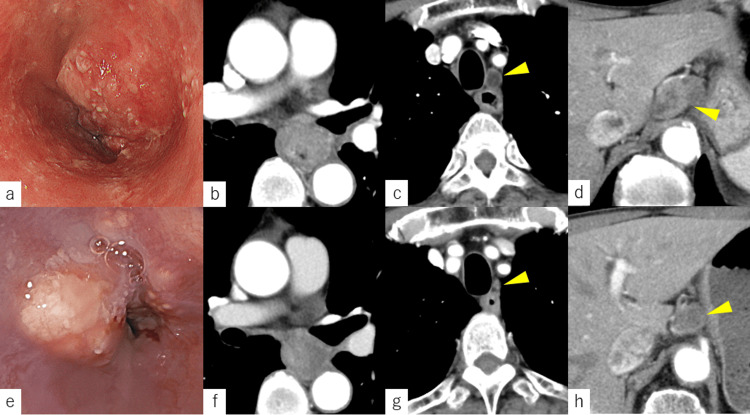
Images from upper gastrointestinal endoscopy and computed tomography (CT) Before NAC: (a) Upper gastrointestinal endoscopy shows an advanced tumor with stenosis; (b) CT shows the advanced cancer in the middle thoracic esophagus; (c) CT shows the lymph node metastasis around the left recurrent laryngeal nerve; (d) CT shows the abdominal lymph node metastasis. After NAC: (e) Upper gastrointestinal endoscopy shows that the stenosis remains; (f) CT shows that the tumor appears decreased; (g) CT shows that the lymph node metastasis around the left recurrent laryngeal nerve is significantly smaller; (h) CT shows that the abdominal lymph node metastasis has shrunk slightly.
